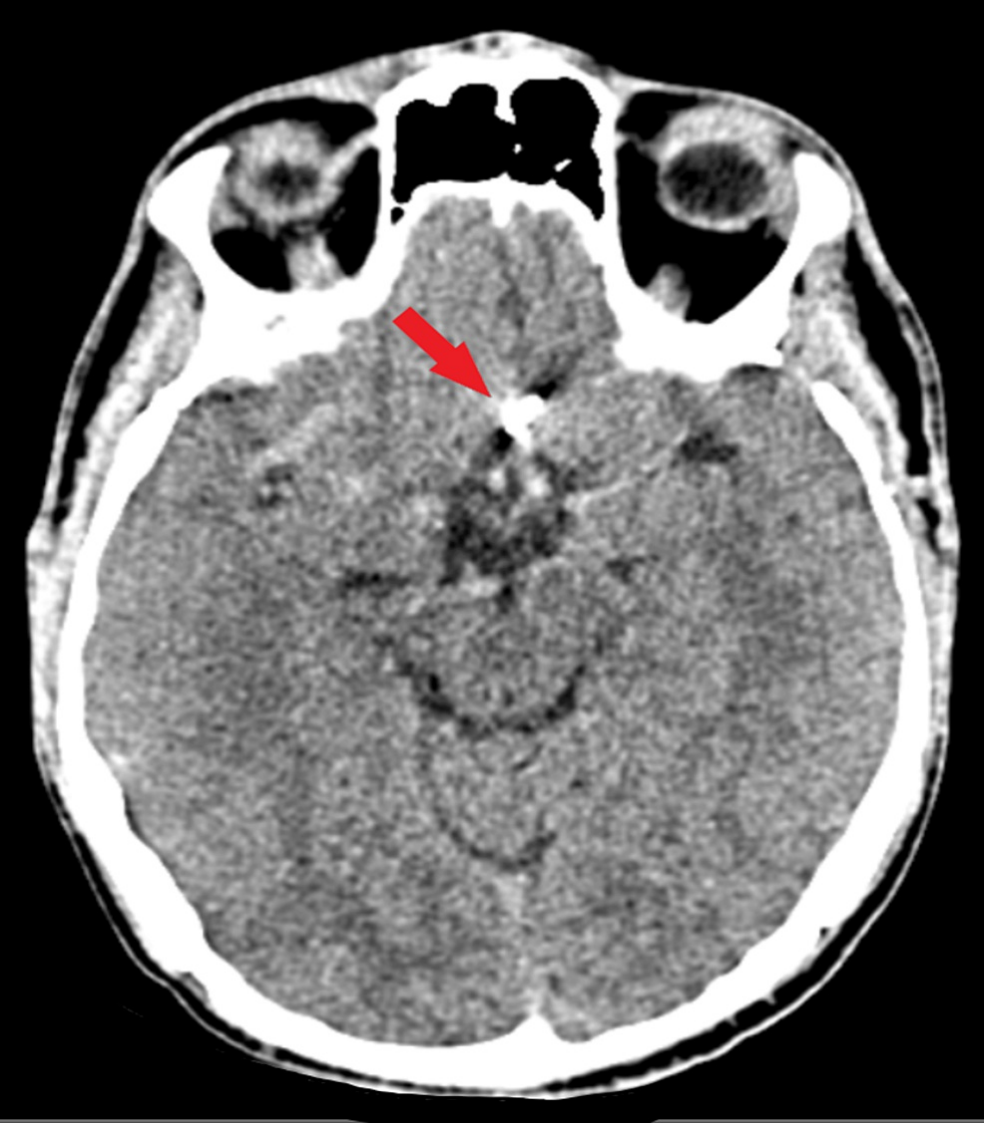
Follow-up CT scan. Axial section of a five-year follow-up CT examination, revealed the absence of an intracranial hemorrhage, with aneurysm clip present (red arrow).
